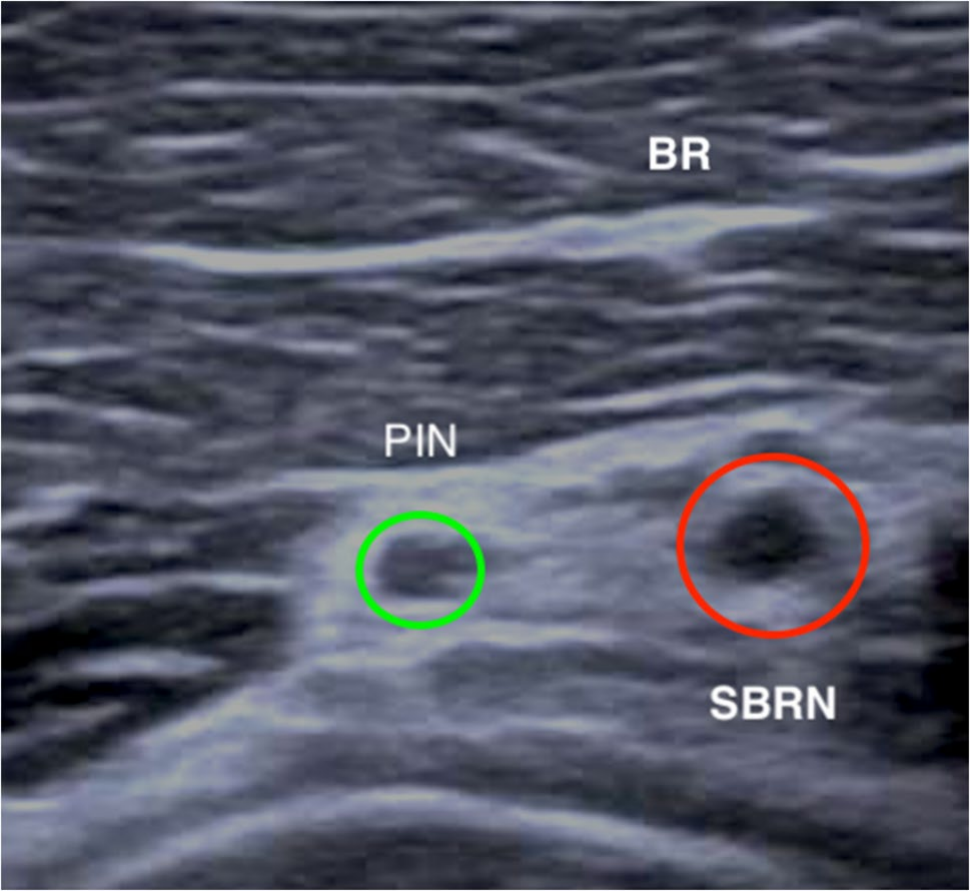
Ultrasound image of the compression site, showing an increased cross-sectional area and hypoecogenicity in the SBRN, as signs of a focal nerve compression. BR = Brachioradialis; PIN = Posterior interosseous nerve; SBRN = Sensory branch of the radial nerve
